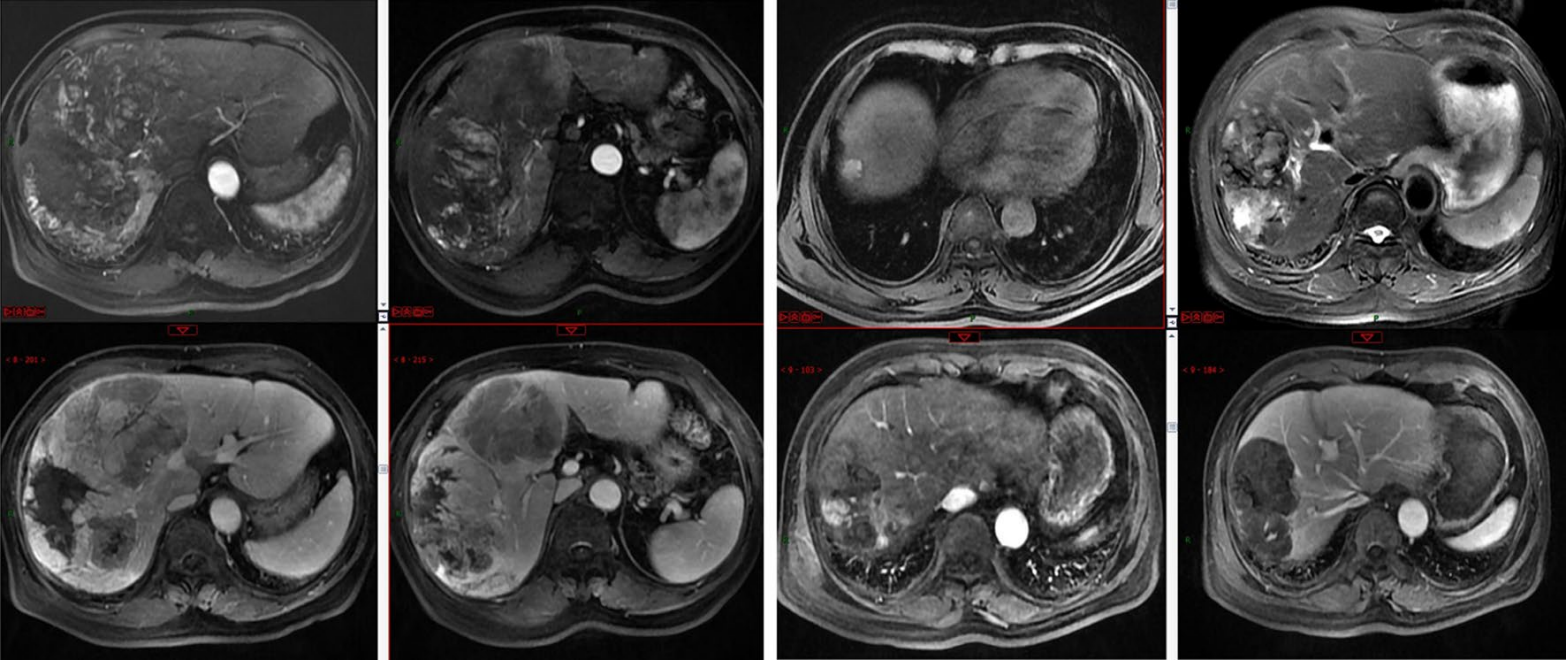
MRI image of a patient with stage IIIb HCC who achieved PR after conversion therapy. First Row (Pre-treatment MRI Scans): First: Pre-treatment axial MRI scan showing a large intrahepatic lesion with arterial phase hyperenhancement. Second: Venous phase MRI scan before treatment, highlighting the extent of the lesion with venous phase hyperintensity and the relatively decreased portal supply. Third: Delayed phase pre-treatment MRI scan, indicating a lesion with delayed phase fast-out intensity. Fourth: T2-weighted MRI scan before treatment, with the portal vein tumor thrombosis exhibiting high signal intensity. Second Row (Post-treatment MRI Scans): First: Arterial phase MRI scan after initial conversion therapy, demonstrating decreased lesion enhancement. Second: Venous phase MRI post-conversion therapy, showing partial response with reduced venous phase hyperintensity and the recovery of portal supply. Third: Delayed phase MRI after conversion therapy, revealing a decrease in lesion size with delayed phase hyperintensity. Fourth: T2-weighted MRI scan post-conversion therapy, showing a reduction in lesion size and elimination of portal vein tumor thrombosis.
